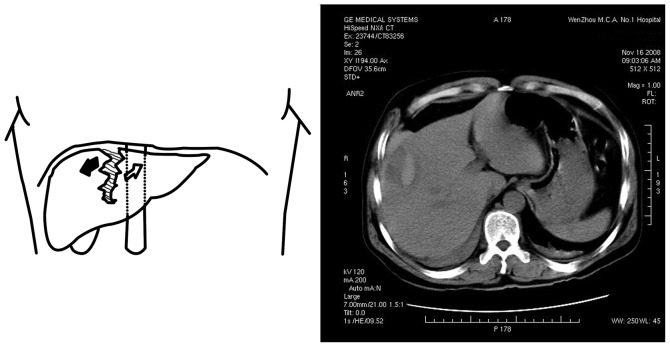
When the liver is propelled in an anterior-posterior direction, the retrohepatic vena cava fixes the liver (empty arrow), and the liver lacerates along Cantlie’s line due to the acceleration of the right lobe of the liver (black arrow). CT film shows a hepatic injury caused in this way by a traffic accident.
